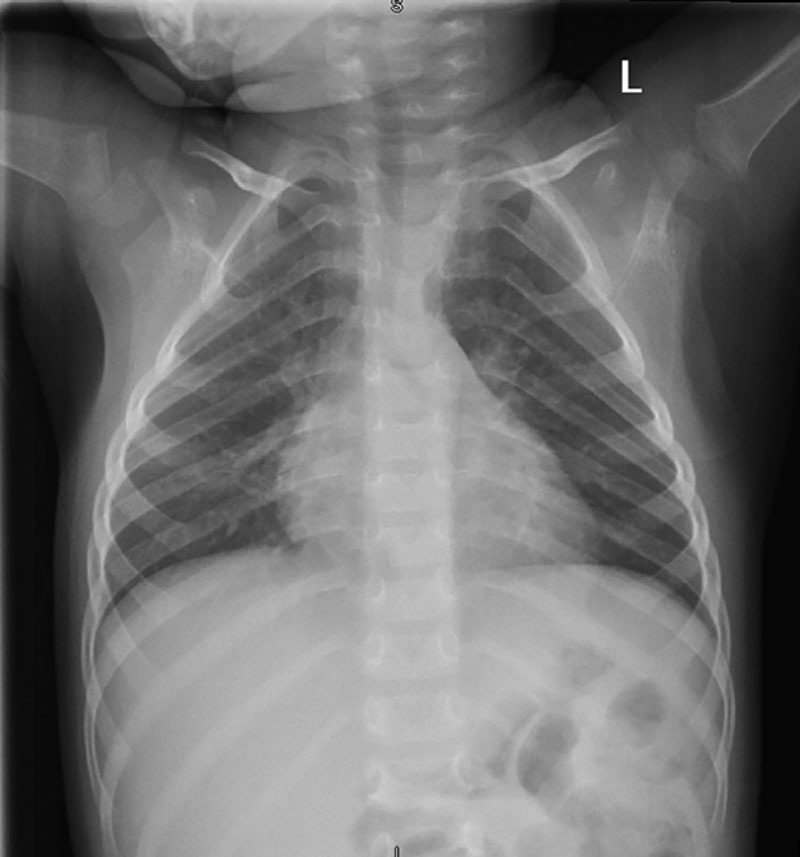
Plain anterior-posterior chest radiograph demonstrating bilateral perihilar interstitial markings.
